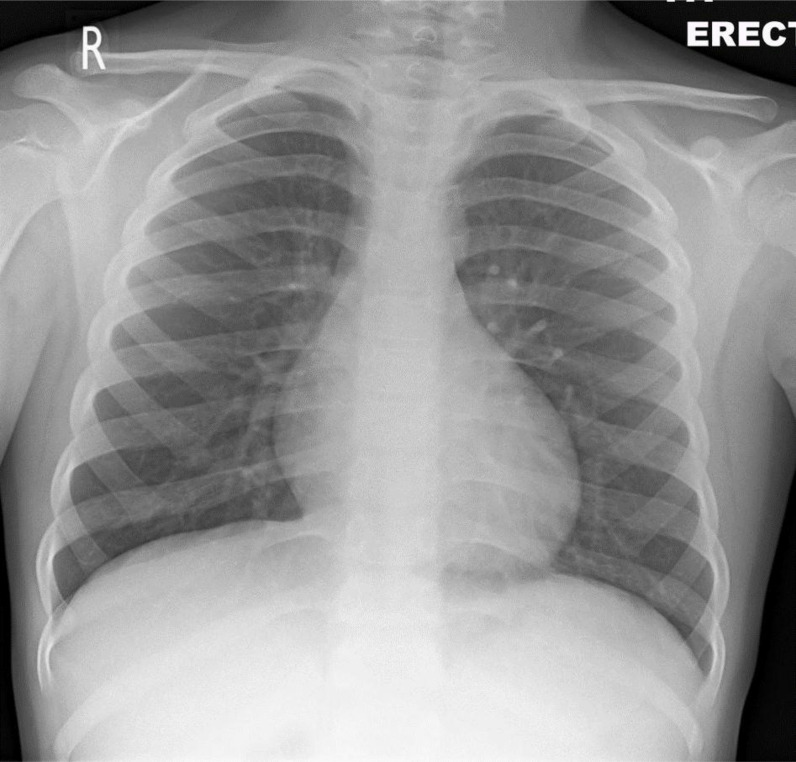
Grade 0 chest X-ray: no abnormal radiological findings
